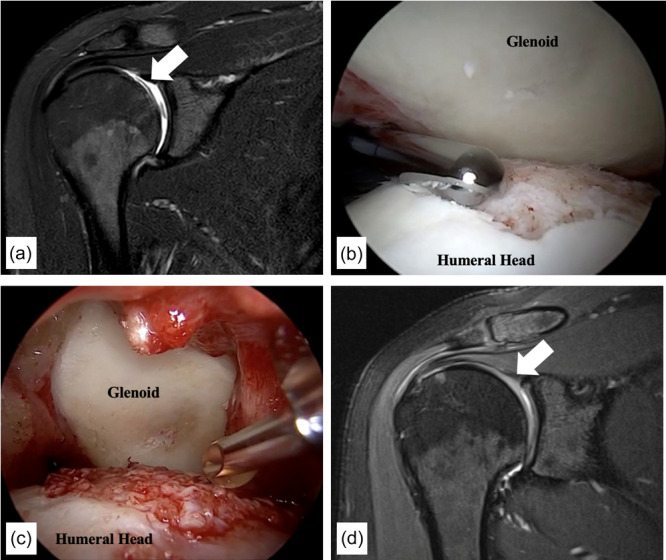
Preoperative MRI scan in the coronal plane of Patient ID 8 showing a cartilage defect at the humeral head (a). Intraoperative images of the same patient demonstrate the cartilage defect prior to debridement (b) and after MCI (c). The MRI after 27 months shows successful defect coverage (d). MCI, minced cartilage implantation; MRI, magnetic resonance imaging.
